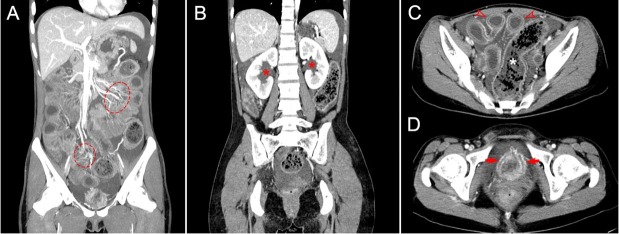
Computed tomography (CT) imaging features of lupus enteritis and cystitis. A: Coronal CT image shows mesenteric vessel engorgement, manifesting as the “comb sign”(dotted circle), a characteristic finding of mesenteric inflammation; B: Coronal CT image reveals renal pelvic dilation (red asterisk), indicative of hydronephrosis, with findings raising concern for urinary tract involvement; C: Transverse CT image demonstrates small bowel wall thickening exhibiting the “target sign”(hollow red arrow), accompanied by luminal dilation, intraluminal fluid accumulation, and ascites. Additionally, there is marked colonic and sigmoid dilation with associated wall thinning (white asterisk); D: Transverse pelvic CT image shows bladder wall thickening with edema (red arrow), characteristic of lupus cystitis.
